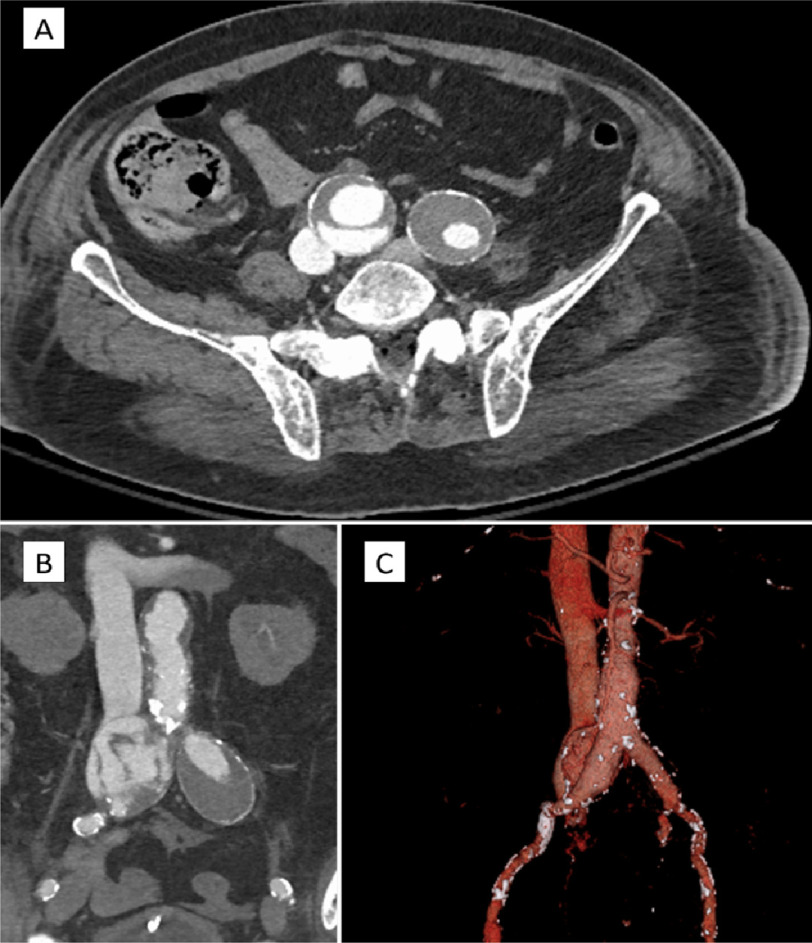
CT-Angiogram images demonstrating the fistulous communication between the right common iliac artery and the inferior vena cava. The axial (A), coronal (B), and 3D reconstructed (C), display the fistulous communication with radiographic dye traversing the defect. Images courtesy of the University of Louisville, Department of Radiology (Dr. Mohammad Ismail).
